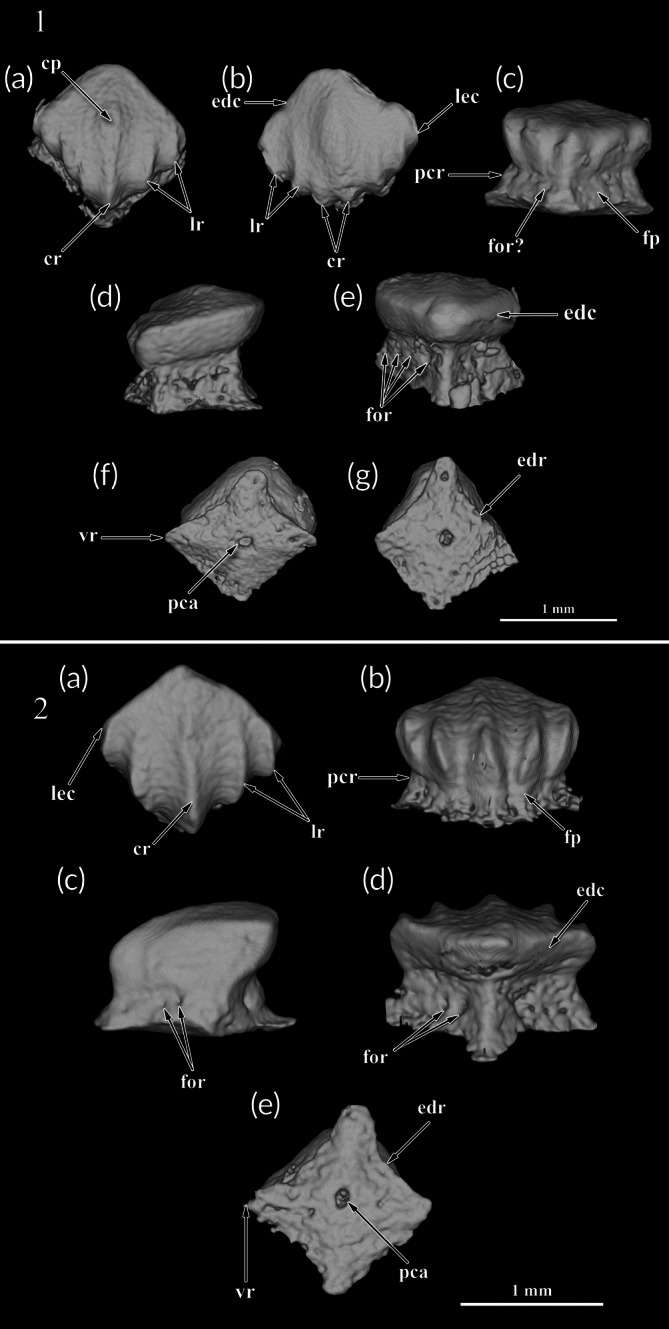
1 = Dorsal type 1 denticles (UFPB 14343 female, 1068 mm PCR). (1a) D1 dorsal view. (1b) D2 dorsal view. (1c) D1 frontal view. (1d) D1 lateral view. (1e) D2 posterior view. (1f) D1 ventral view. (1g) D2 ventral view. In (1a, 1b, 1f, 1g) anterior to bottom; In (1c) anterior to front; In (1d) anterior to left; In (1e) anterior to back. 2 = Dorsal type 2 denticles (UFPB 14343 female, 1068 mm PCR). (2a) D3 dorsal view. (2b) D3 frontal view. (2c) D3 lateral view. (2d) D3 posterior view. (2e) D3 ventral view. In (2a, 2e) anterior to bottom; In (2b) anterior to front; In (2c) anterior to left; In (2d) anterior to back (micro CT‐scan).
